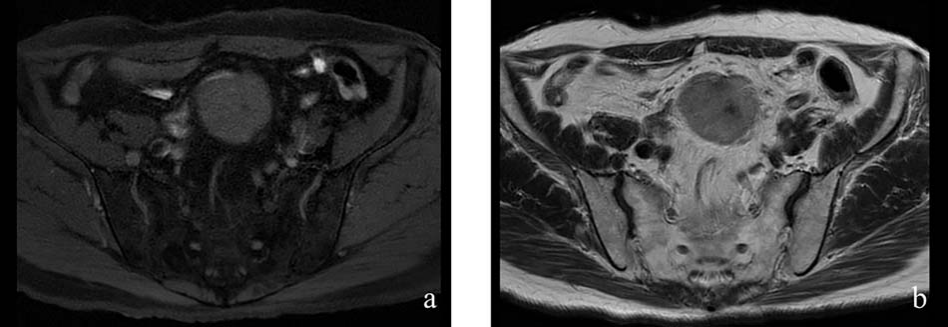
Figure 2: (a, b) The tumor had hypointensity on the T1-weighted images and mild hyperintensity on the T2-weighted images.
